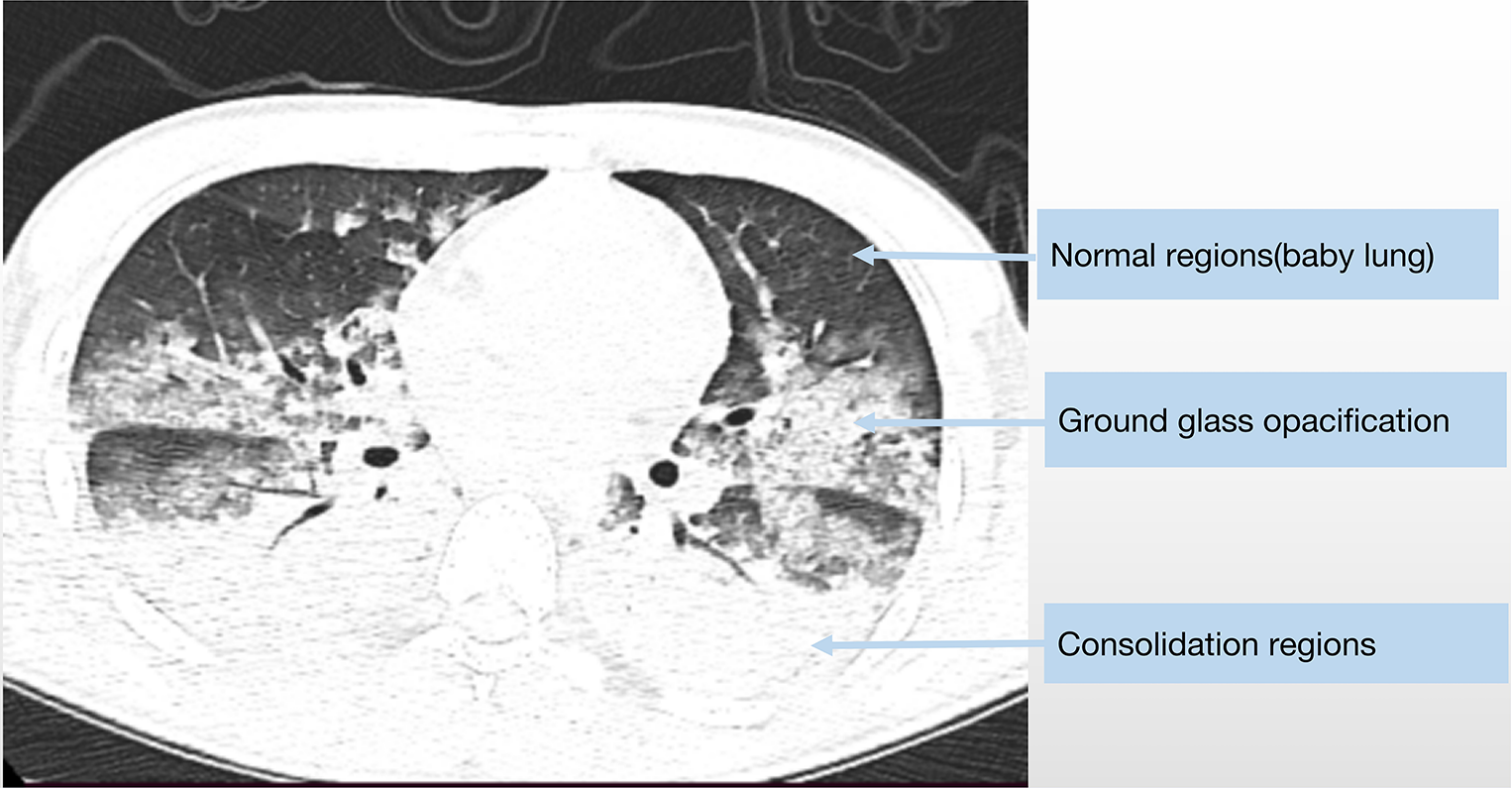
The chest CT scan of a 19-year-old male diagnosed with fulminant myocarditis show similar radiologic characteristics as classic ARDS.
